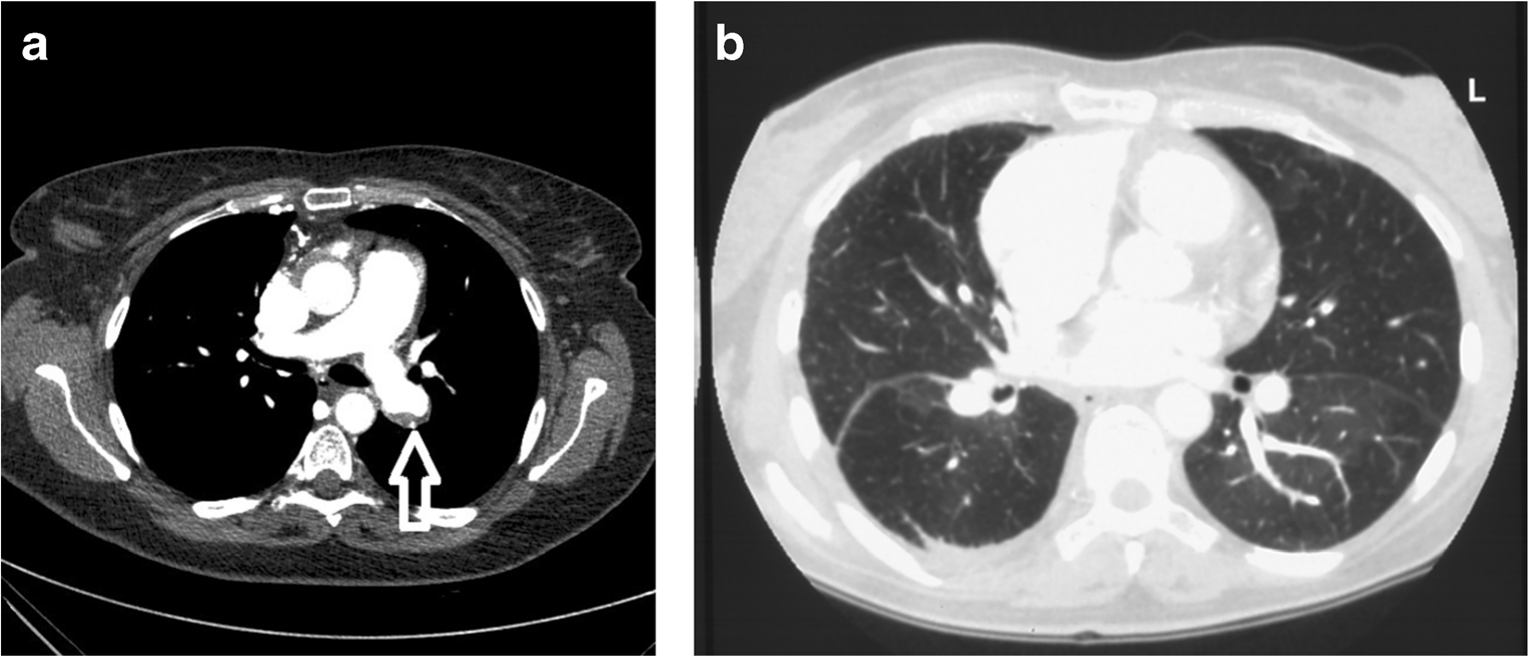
a Contrast-enhanced chest CT in CTEPH shows eccentric thrombotic material within the left pulmonary artery (*arrow*). b Contrast-enhanced chest CT scan in CTEPH before pulmonary endarterectomy showing mosaic perfusion
